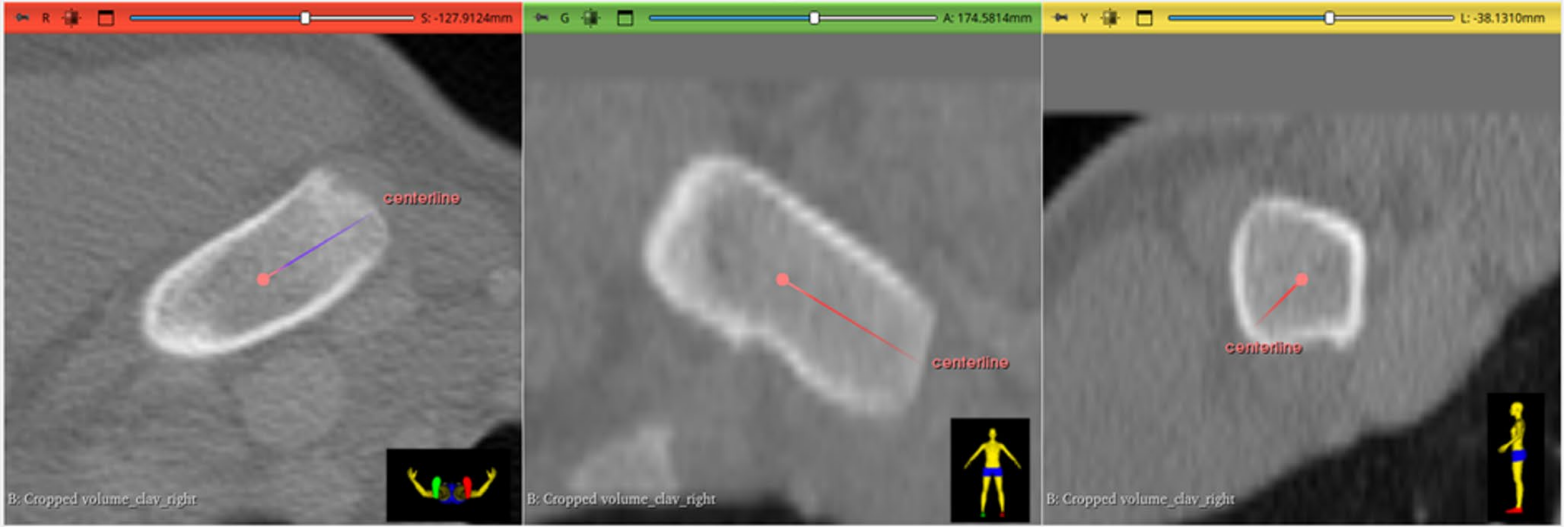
The central position of the distal endpoint of the centerline within the metaphysis was verified in all three CT image planes. (red: axial view, green: coronal view, yellow: sagittal view)
